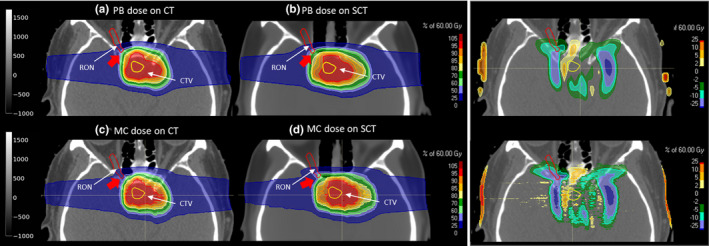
Pencil beam (PB) doses for patient #1 on the computed tomography (CT) (a) and synthetic CT (b), together with the dose difference, and the corresponding Monte Carlo (MC) doses (c and d). The right optic nerve (RON) and the clinical target volume are contoured in red and yellow, respectively. The red arrow points toward the region next to the nasal cavity, where a big discrepancy between pencil beam and MC doses in the sCT is found for the RON D_2_.
